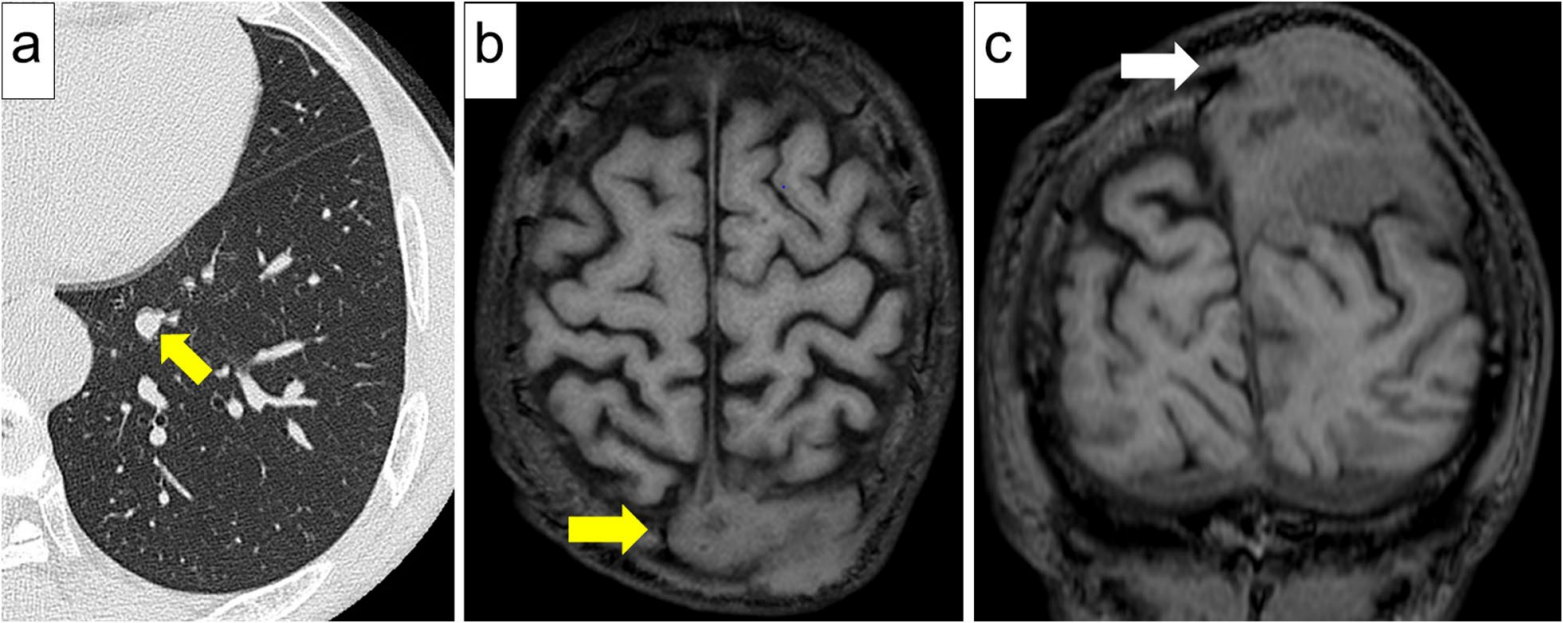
Medical imaging findings. a Computed tomography of the chest showing a 10-mm solitary pulmonary nodule in the left lower lobe of the lung (yellow arrow). b Two years before the referral, magnetic resonance imaging revealing a 65-mm tumor in the left parietal region (yellow arrow, axial section) and c skull infiltration of the tumor (white arrow, coronal section)
